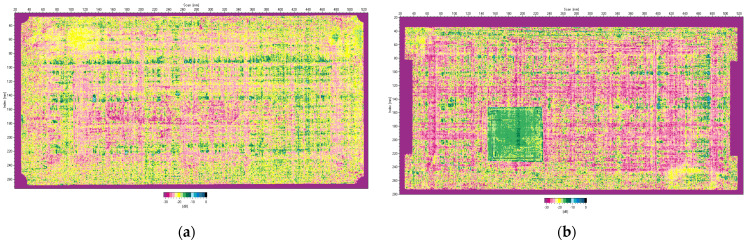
Ultrasonic C-scan (time-of-flight mode) images of the plates: (a) healthy plate; (b) plate with an artificial delamination.
